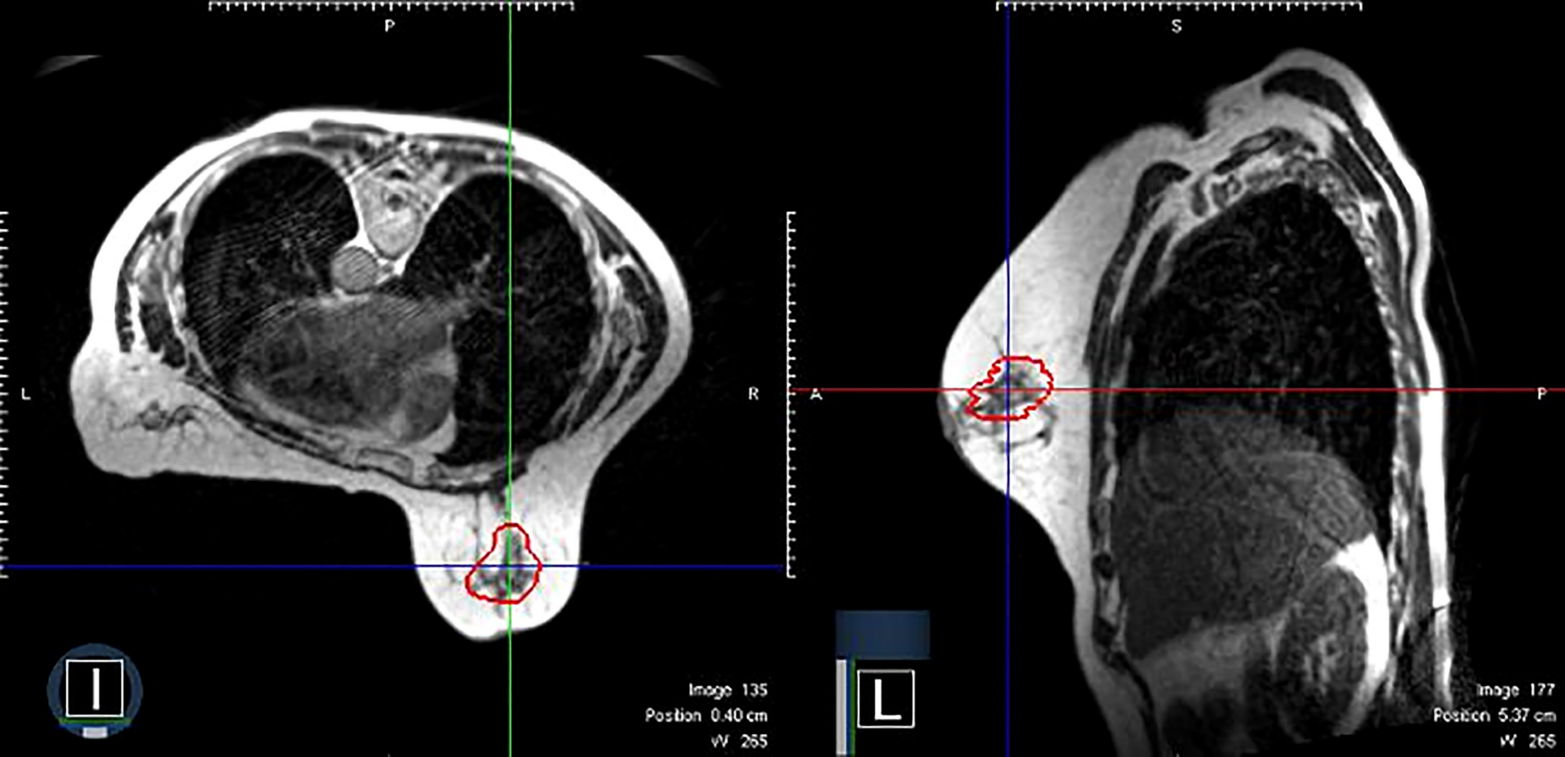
An example of clinical MRI-Linac images utilized for therapy guidance. The panel on the left shows a patient receiving prone breast irradiation with an MRI image in the axial plane. The same patient with a sagittal plane image. The red contour depicts the lumpectomy surgical cavity which serves as the clinical target volume.
